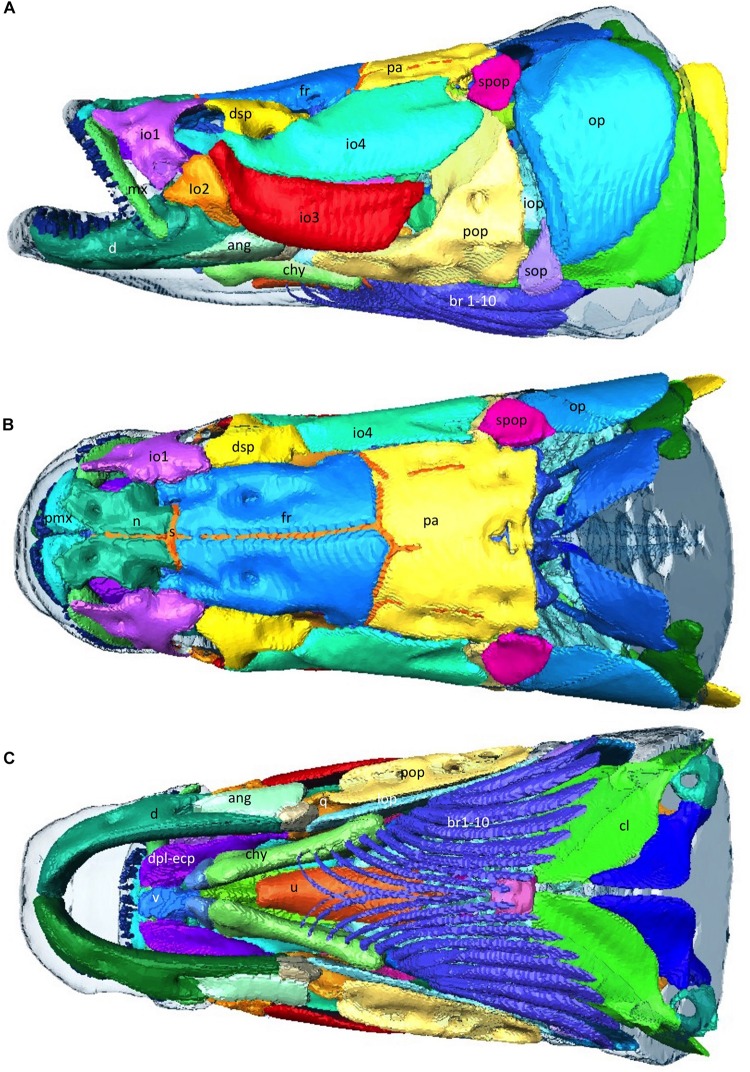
Musculoskeletal -Head. (A–C) 3D reconstructions from CT data, of the external bony armor plates of the head with integument overlain as transparent surface. Abbtp, anterior basibranchial toothplate; ang, angular; ant, antorbital; br, branchiostegal rays; chy, Ceratohyal; cl; d, dentary; dsp, dermosphenotic; fr, frontal; io 1–4, infraorbital 1–4; iop, interopercular; iop, infraopercular; mx, maxilla; n, nasal; op, opercle; pa, parietal; pmx, premaxilla; pop, preopercle; q, quadrate; s, suture; sop, subopercle; spop, suprapreopercle; u, urohyal; v, vomer. (Bones referenced as per Steward 2013).
